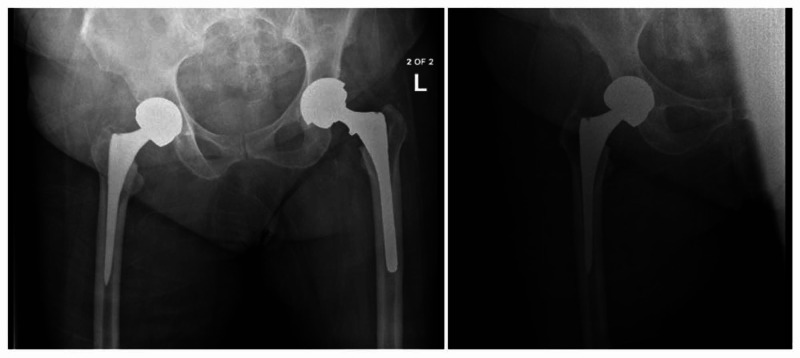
Preoperative plain radiograph to assess symptomatic right primary total hip arthroplasty (THA) that was performed by another surgeon.
